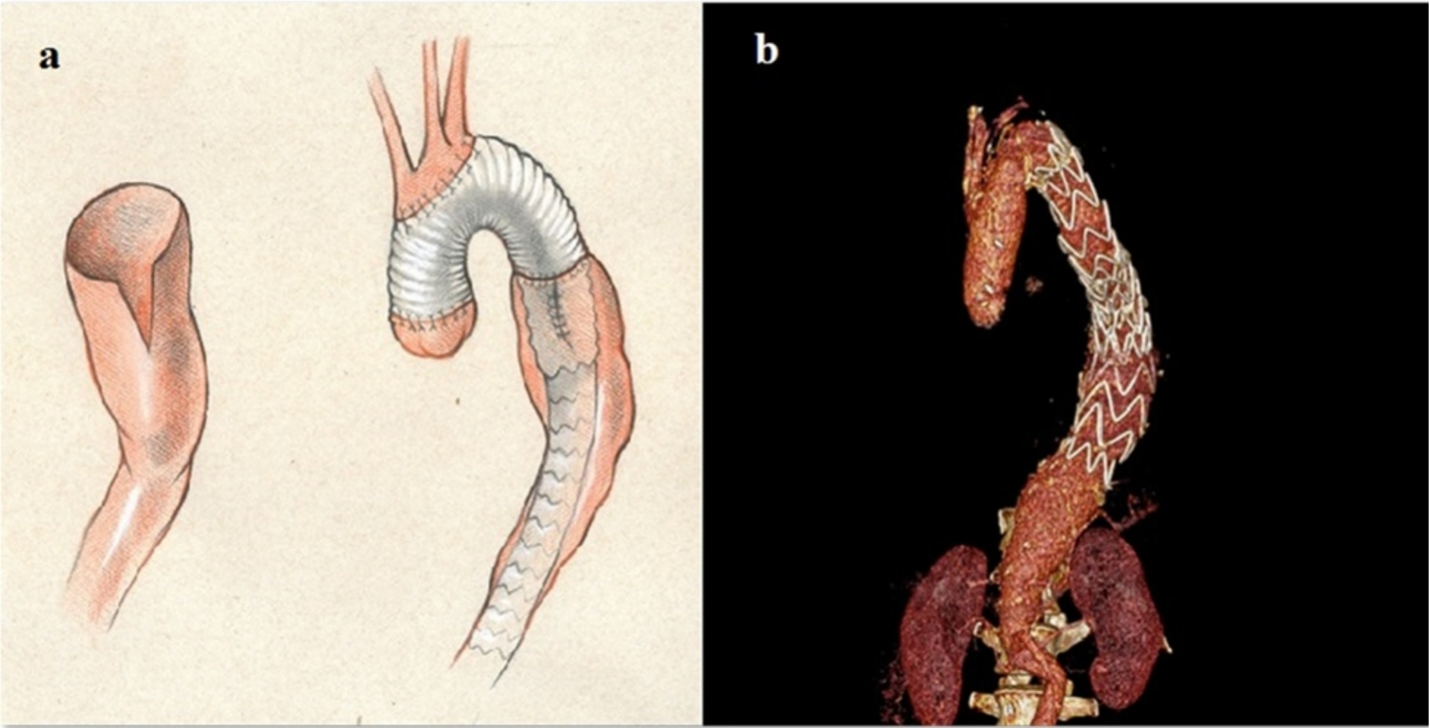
Animation and postoperative image. Animation of “*V*” descending aortoplasty (on the anterior wall of the proximal descending aorta a V-shaped aortic wall resection of 5 cm in length and 3 cm in width was done and the defect on the aortic wall was primarily closed with a suture after the elephant trunk had been inserted into the descending aorta [a]). Postoperative image of the patient’s final aortic treatment (CT scan [b]).
